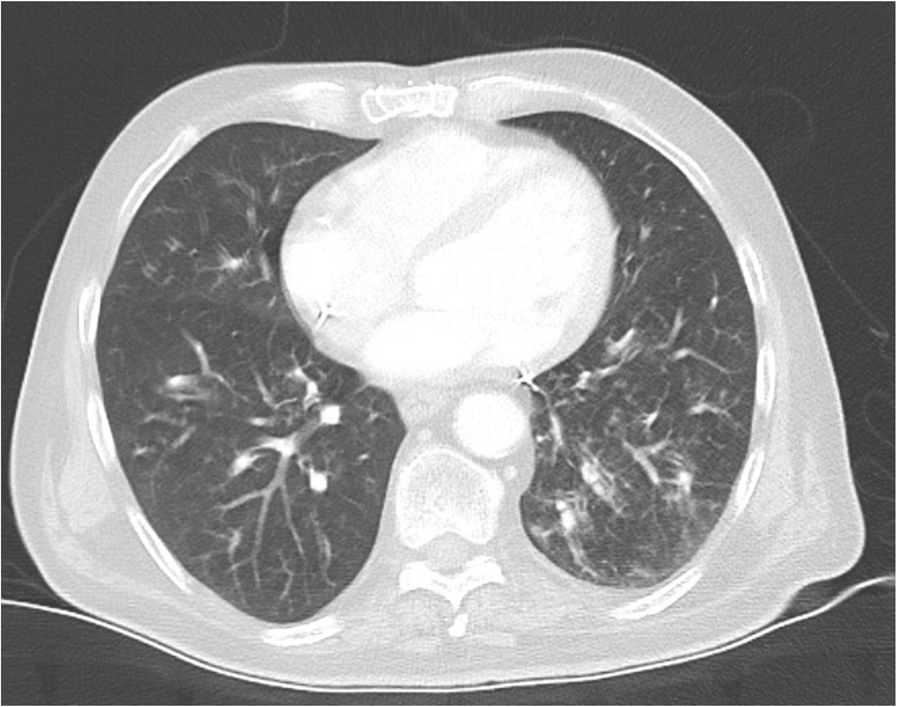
Axial window CT-scan on day 1 showing pneumonitis with tree-in bud pattern and ground glass opacities
